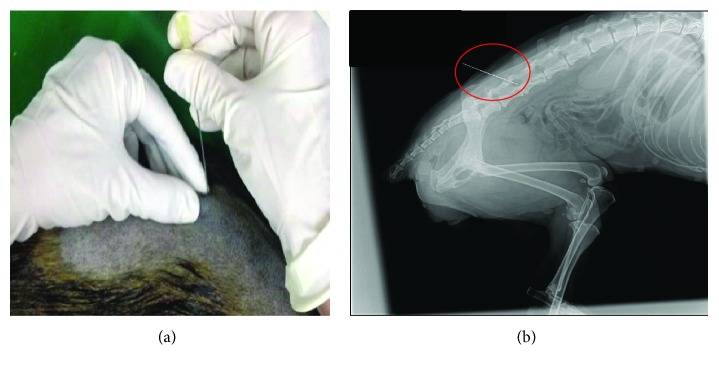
Localization of the injection site. (a) Photograph depicting the procedure of percutaneous injection. (b) X-ray image of the vertebral column, highlighting the affected intervertebral space.
